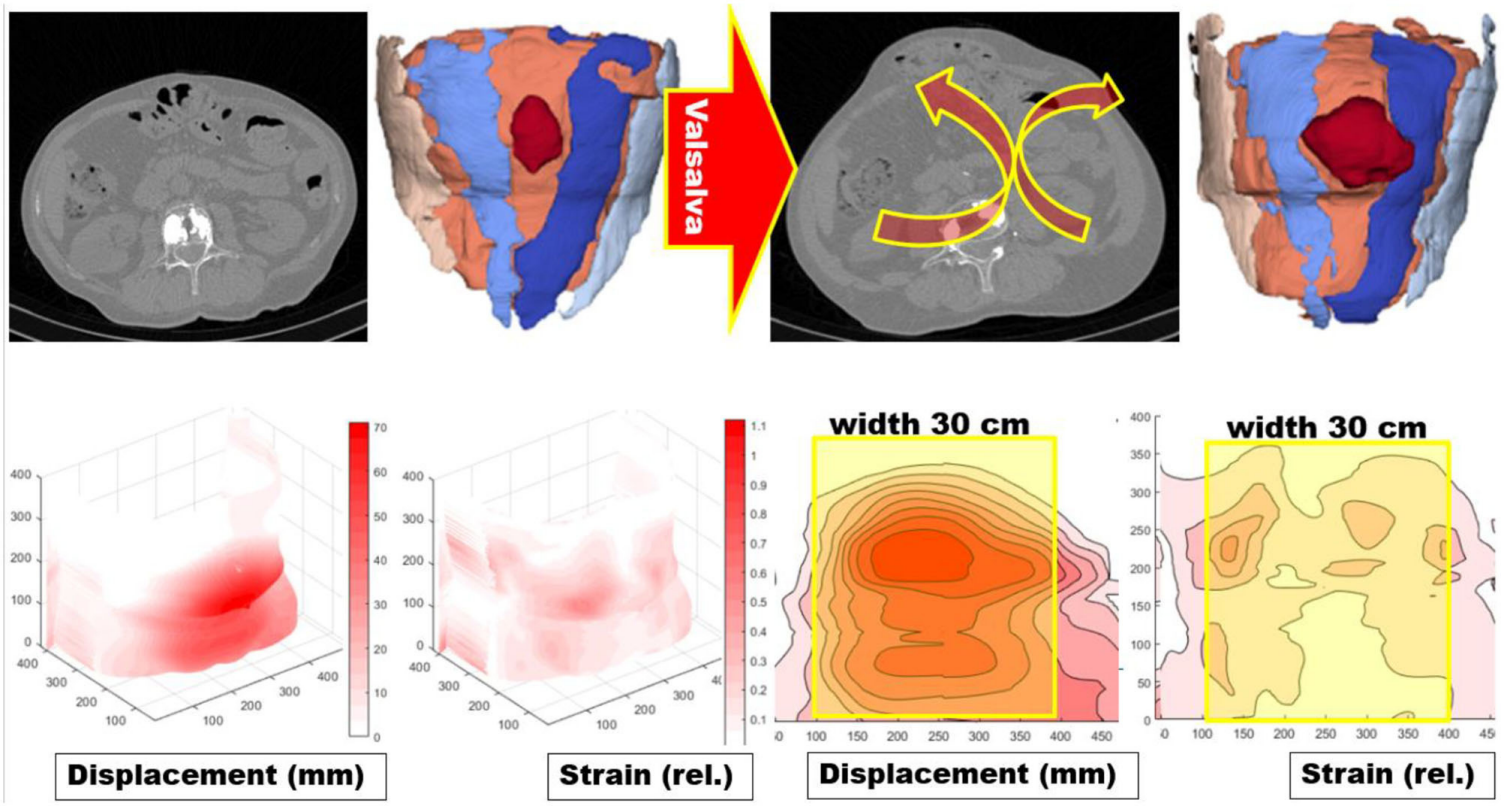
(Top from left to right) a scan central to the incisional hernia of a CT abdomen at rest, analysis of the data with artificial intelligence giving the rectus muscles as blue areas and marking the hernia with a dark red color (10). The application of Valsalva's maneuver unmasks a regional instability of the abdominal wall with an outward motion marked with the red arrows with the yellow borders. The resulting hernia orifice enlarges and changes its shape. (Bottom from left to right) Surface displacement of the abdominal wall during Valsalva's maneuver in millimeters and the resulting strain field in 3 D and as a planar projection (11). On the planar projections, a hernia mesh 30 cm wide and 45 cm long is superimposed with the center at the umbilicus. It was evident that stress concentrations and peaks of distortions can occur at the edge of the mesh resulting in an unstable reconstruction.
